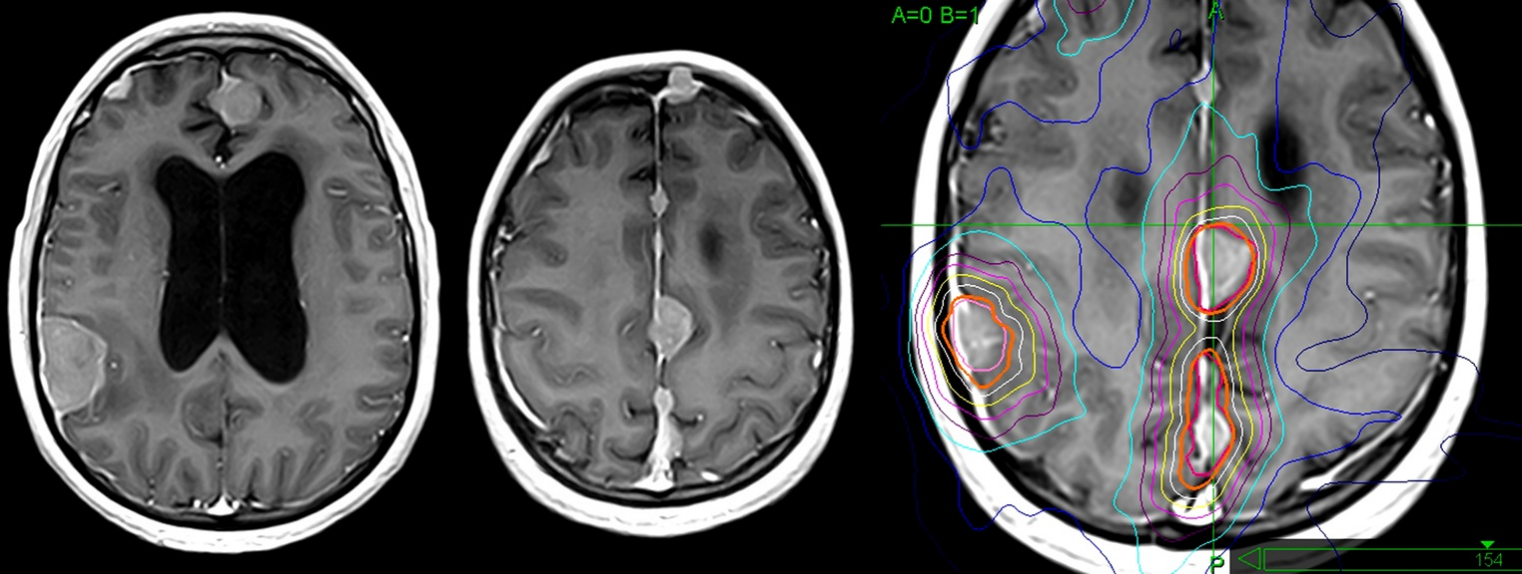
On MRI obtained 7 months after radiation treatment of skull base metastasis multiple leptomeningeal metastatic tumors are detected Left: pre-treatment MRI Right: dose plan of CyberKnife radiotherapy to the new leptomeningeal metastases (February, 2013) Lines: thick orange line - prescribed isodose, red and light pink lines - tumor contours. Isodose lines: white - 70%, yellow - 60%, magenta - 50%, purple - 40%, blue - 30%, dark blue - 10%
